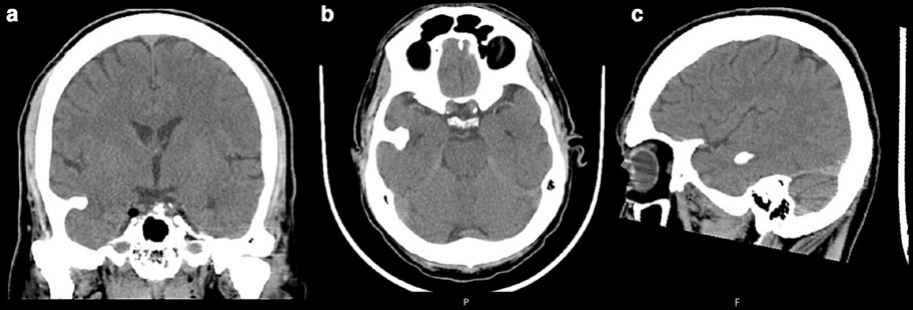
Brain CT showing no parenchymal compromise in coronal (A), axial (B) sagittal and (C) planes
